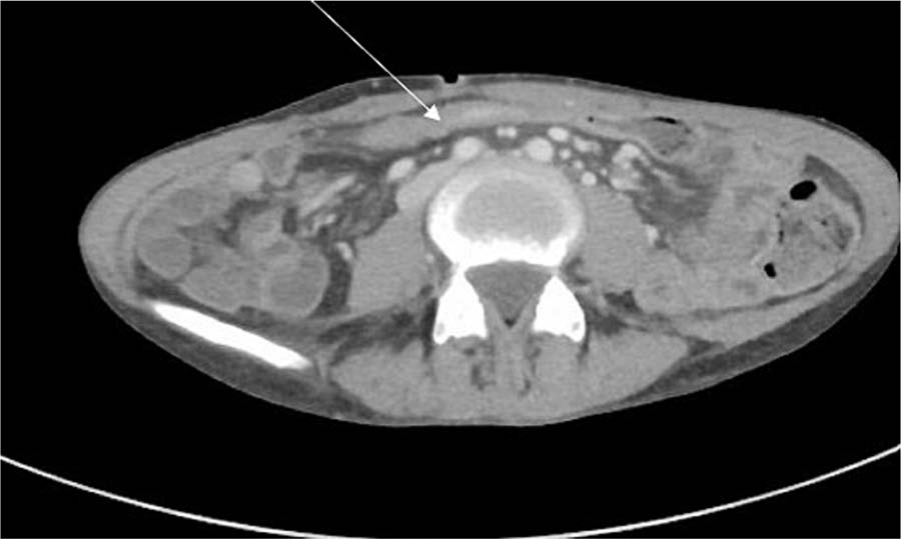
CT scan demonstrating thickening and abnormal enhancement of the neoterminal ileum (arrow), consistent with active CD.
